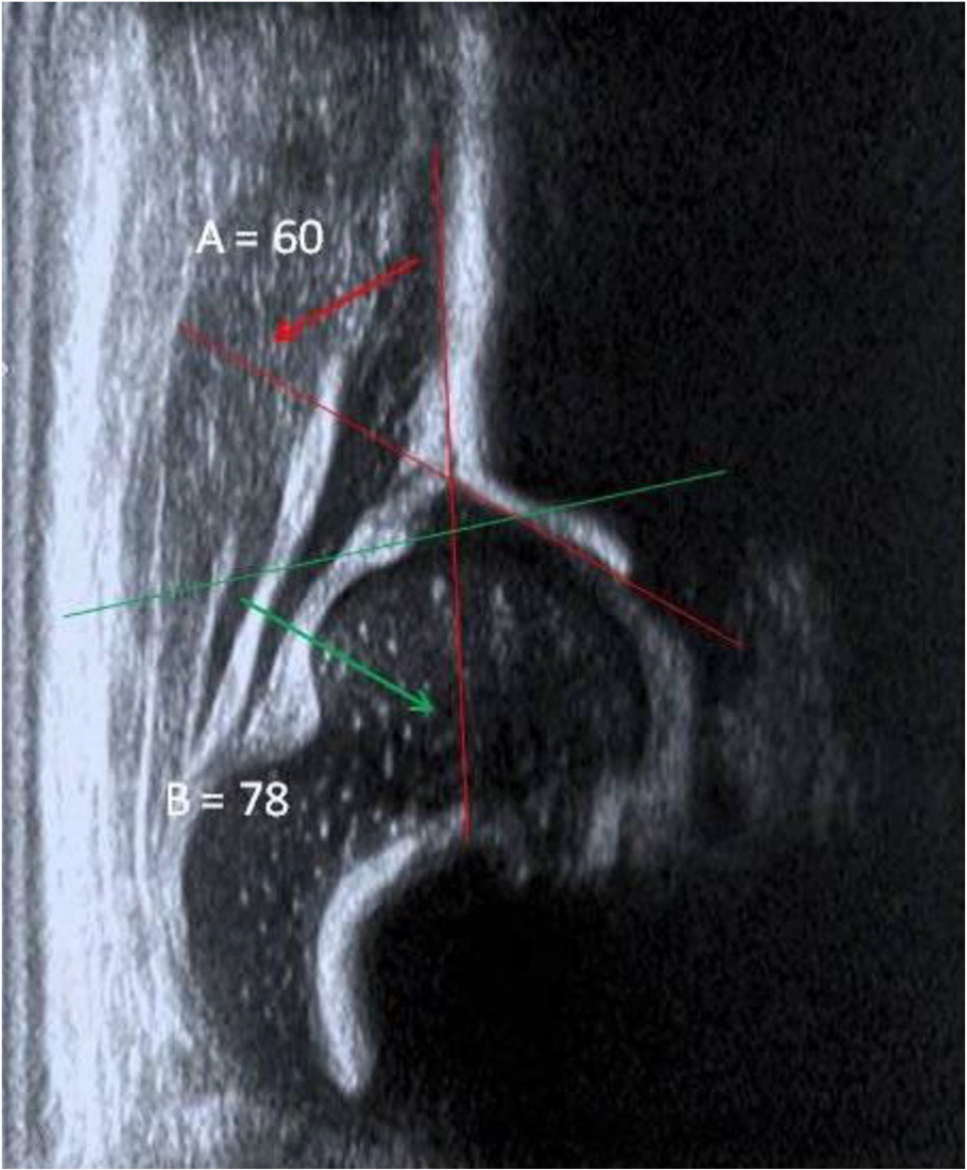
Graf’s method for ultrasound evaluation of developmental dysplasia of the hip shows a normal hip. Case courtesy of Mr Sandeep Hemmadi, Radiopaedia.org, rID: 45301
